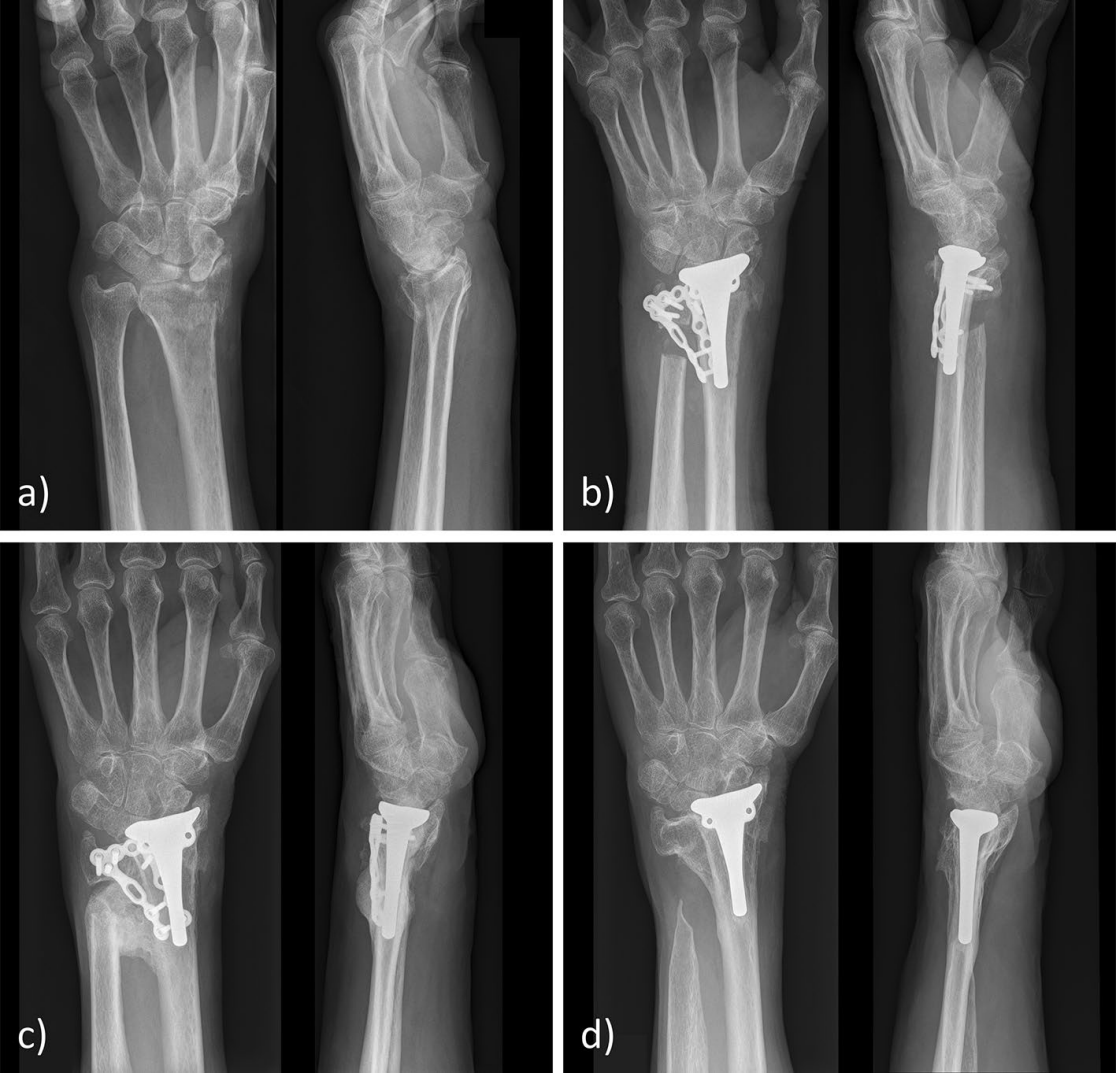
a Radiograph of the initial fracture. b Radiograph after hemiarthroplasty and Sauvé-Kapandji procedure with dorsal plating. c Severe heterotrophic ossifications 4 months after surgery leading to significant functional impairment. Revision included excision of the ossifications, removal of the dorsal radioulnar plate and a pronator quadratus interposition. d Radiograph at the follow-up 34 months after revision
